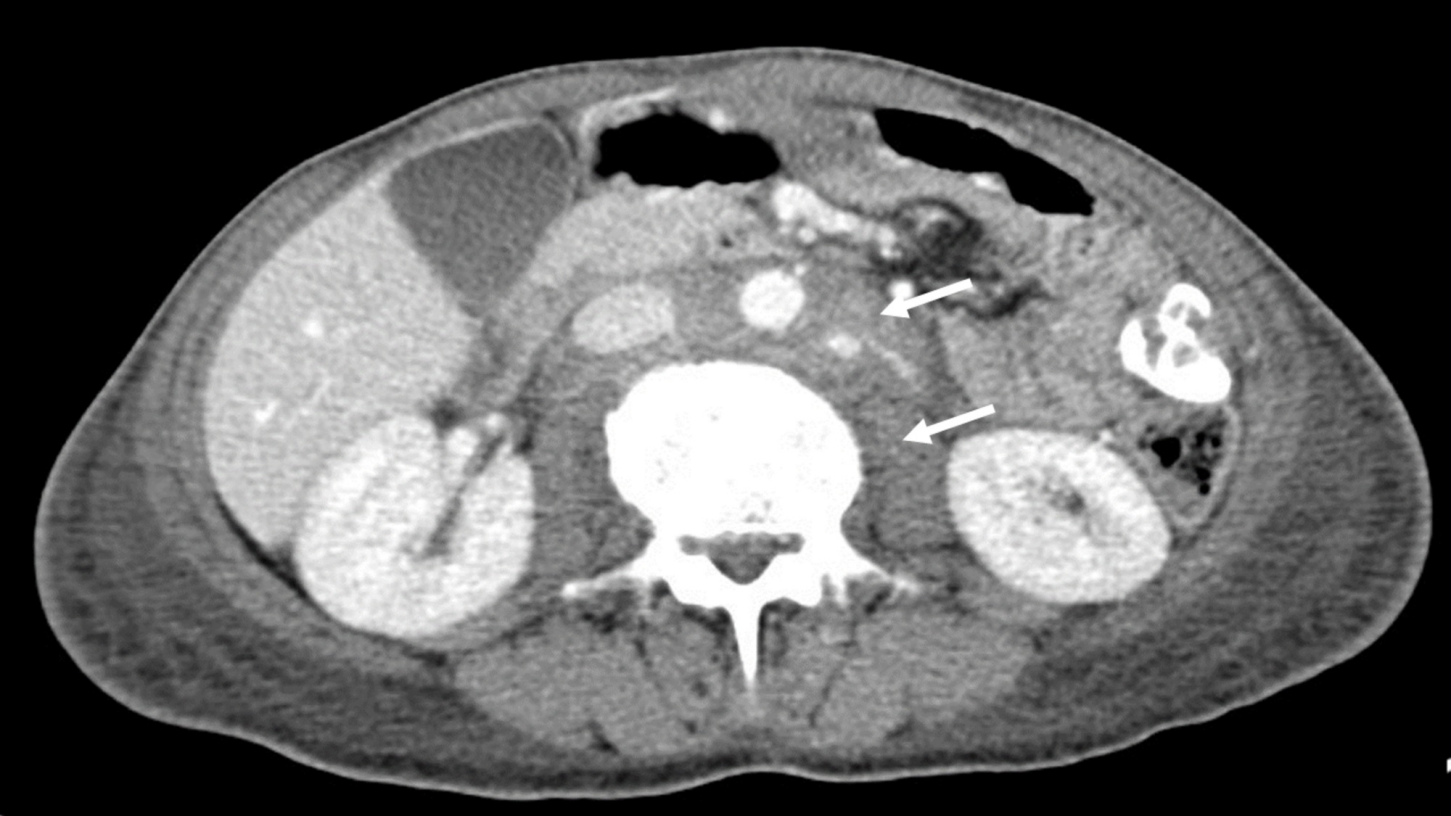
CT of the abdomen showing conglomerate of adenopathies
